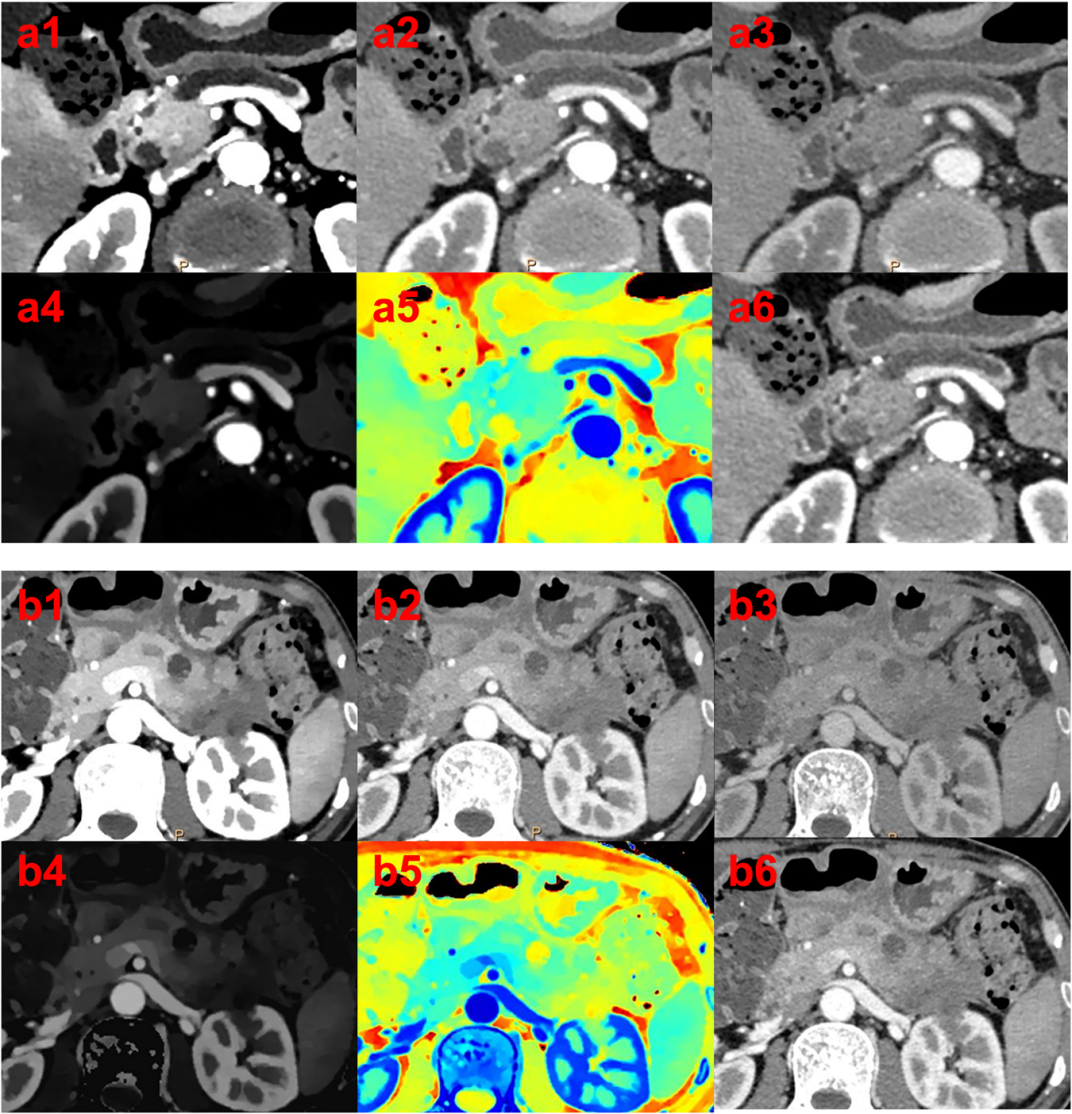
Examples of PDAC patients with high/low TMB. The a (1–6), b (1–6) Images are VMIs at 40, 70, and 100 keV, IC maps, Zeff images, and conventional CT images. a(1–6) A 66-year-old female was diagnosed with PDAC, and the TMB of the tumour was 12.07 mut/Mb. The nICa was 0.137, and the PFS was 14 months. b (1–6) A 40-year-old male was diagnosed with PDAC, and the TMB of the tumour was 0.71 mut/Mb. The nICa was 0.007, and the PFS was 3 months. PDAC, pancreatic ductal adenocarcinoma; TMB, tumour mutation burden; VMIs, virtual monoenergetic images; IC, iodine concentration; Zeff, effective atomic number; nICa, normalised iodine concentration in the pancreatic phase; PFS, progression-free survival
